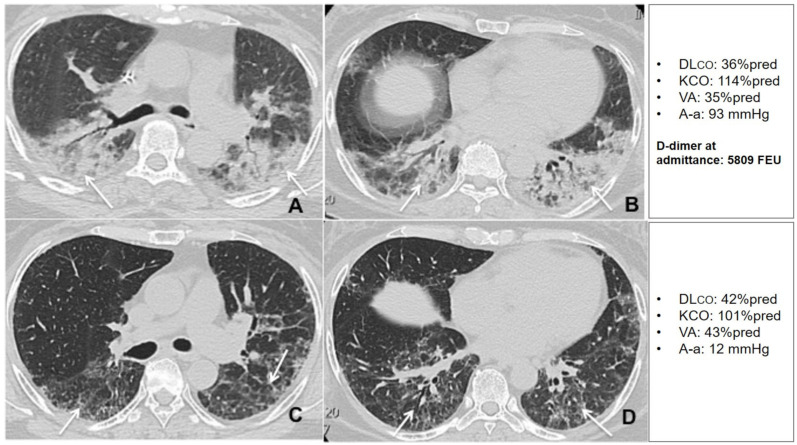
Unenhanced thin section axial (A,B) CT images of the lungs obtained the 12th day post-admission in a 57-year-old woman with arterial hypertension and no previous exposure to cigarette smoke, that was admitted to the HDRU with a PaO_2_/FiO_2_ of 210 mmHg, rapidly worsened her respiratory status and needed invasive mechanical support for 8 days. The images show peripheral posterior GGO and consolidations in both lungs, with predilection for posterior areas (arrows). Unenhanced axial (C,D) CT images of the same patient 6 weeks post-discharge show residual interlobular septal thickening with intralobular lines (arrows) with resolution of previous GGO and consolidation. The patient had a high D-dimer at admission and showed very limited changes in DLco during the recovery period.
